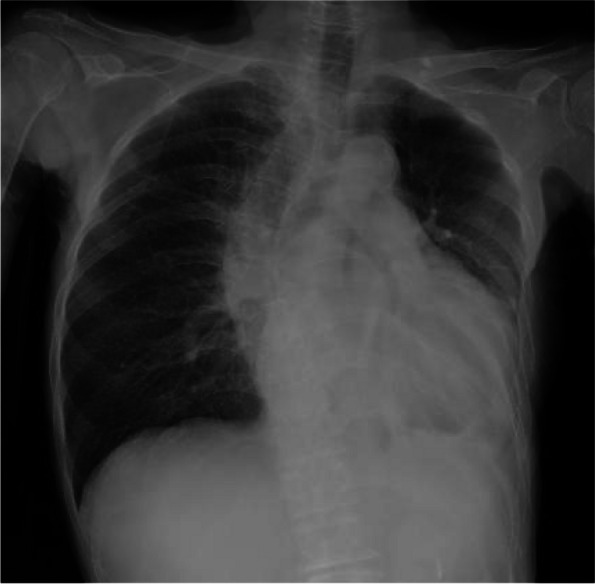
Chest X-ray on admission. Chest X-ray showed scoliosis and cardiac enlargement
